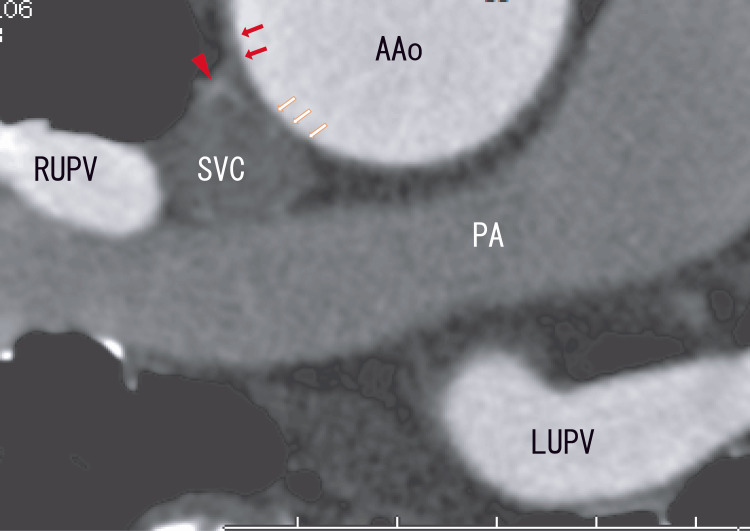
Axial enhanced computed tomography (CT) images showing the AAo and LA Axial enhanced CT images clearly revealed no cleft, no linear white thrombi, and no RUPV thrombi. There were some changes in the walls between the SVC and AAo (red arrows and white arrows). Small normal areas are visible between the white arrows and red arrows. There were white areas in the anterior parts of the SVC (arrowhead), indicating an arterial blood supply. AAo: Ascending aorta; LA: Left atrium; PA: Pulmonary artery; RUPV: Right upper pulmonary vein; SVC: Superior vena cava.
